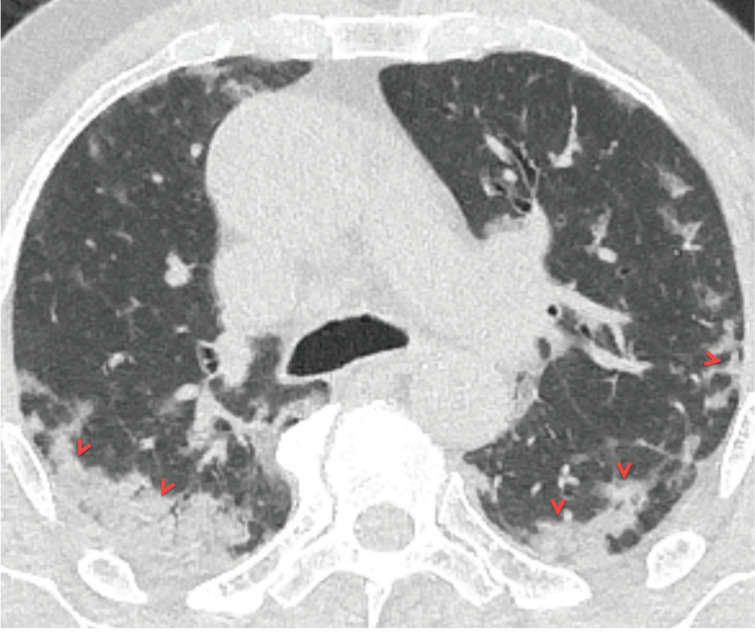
A 66-year-old symptomatic man with COVID-19 pneumonia. Axial CT image at the carina level shows peripheral ill-defined consolidation areas in the both middle-lower lung zones with subpleural involvement (red arrowheads). Note the “air bronchogram sign” within the consolidation areas.
